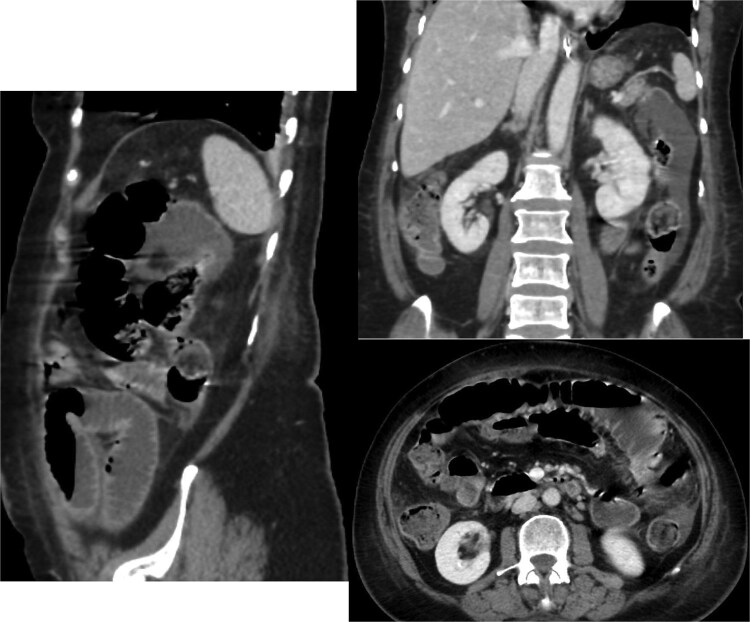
Contrast-enhanced CT scan of the abdomen and pelvis showing the small bowel loops are dilated reaching up to 35 mm. There is a transition zone with 22 mm gallstone in the distal ileum with distally collapsed ileal segments and collapsed large bowel loops representing high-grade obstruction. The gallbladder appears irregular with thickened wall and significant surrounding fat stranding. There is a fistula between the gallbladder and the second part of the duodenum.
